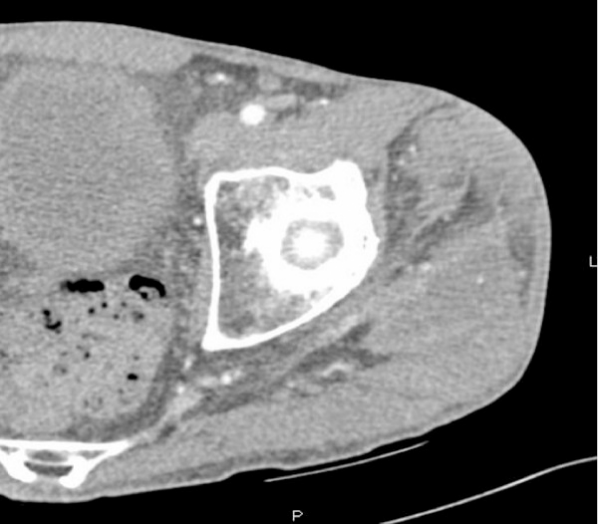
CT scan of left lower extremity showing a 25 mm × 40 mm × 140 mm fluid collection (↔) and peripheral enhancement of the gluteus medius and vastus lateralis muscles.
